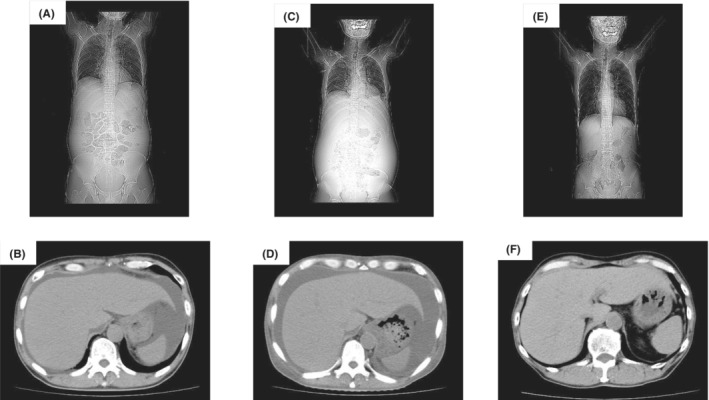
(A, B) Computed tomography (CT) scan showing massive ascites at presentation. (C, D) CT scan taken after G‐CSF for mobilization showing an increase in massive ascites. (E, F) CT scan taken after CART and autologous stem cell transplantation showing improvements.
